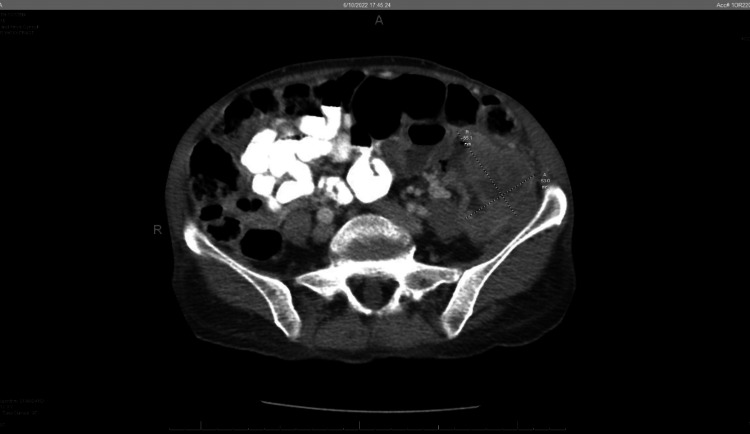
CT A/P demonstrating 65.1 mm x 53.0 mm mass in left sigmoid colon. A/P: abdomen/pelvis
